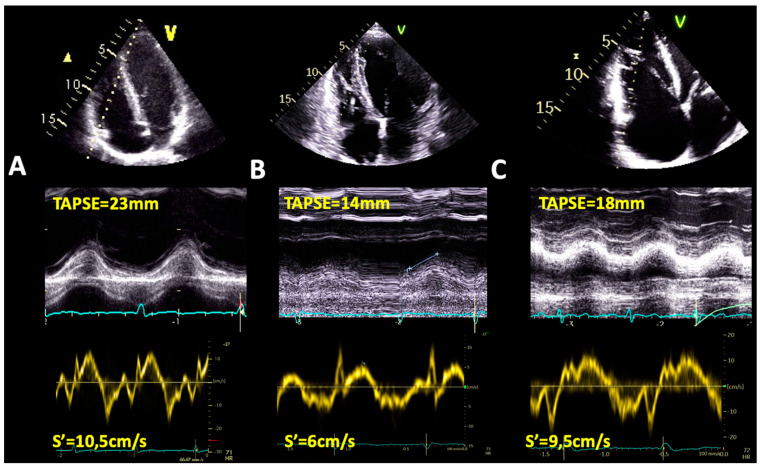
Right ventricular function assessed by M–mode (TAPSE), upper panel, and tissue Doppler imaging (S’ wave velocity), lower panel in (A) a normal subject, (B) a patient with dilated cardiomyopathy and normal right ventricular size and longitudinal dysfunction, and (C) a patient with dilated cardiomyopathy and biventricular involvement, yet normal right ventricular longitudinal function. Abbreviations as in Figure 1.
